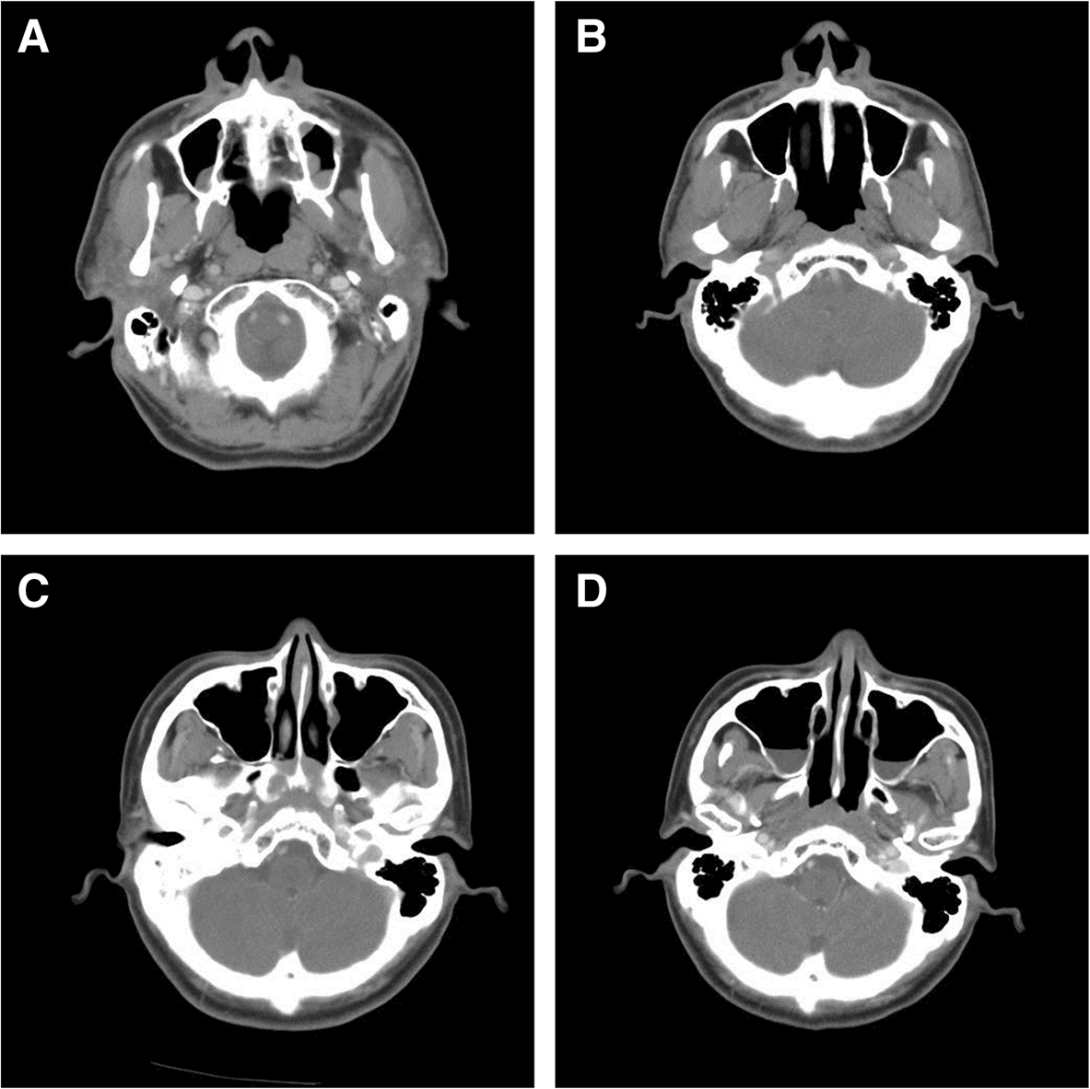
Changes of sinusitis as depicted by the differences between pre-RT and post-RT CT images. a is the pre-RT CT image of a patient who presented with bilateral maxillary sinusitis. b is a follow-up CT image of the same patient taken at 1 year post-RT. As shown, the bilateral sinusitis was diminished. This patient has been disease free for 5 years. c is the pre-RT CT image of another patient showing no evidence of sinusitis. d is a follow-up CT image of the same patient taken at 1 year post-RT. As shown, the patient had developed bilateral maxillary sinusitis at 1 year post-RT, and a biopsy confirmed local recurrence
